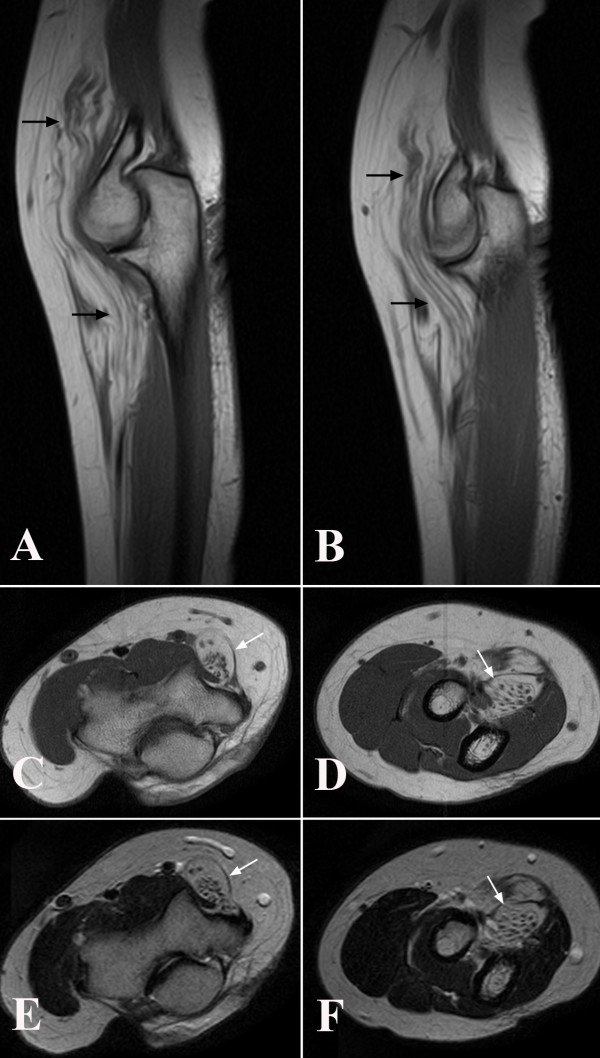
Magnetic resonance investigation (MRI) of the patient showing specific characteristics of a fibrolipomatous hamartoma (arrows) in sagittal sections (T1 weighted in A and B) and in axial sections (T1-weighted in C and D; T2-weighted in E and F) at the elbow region.
